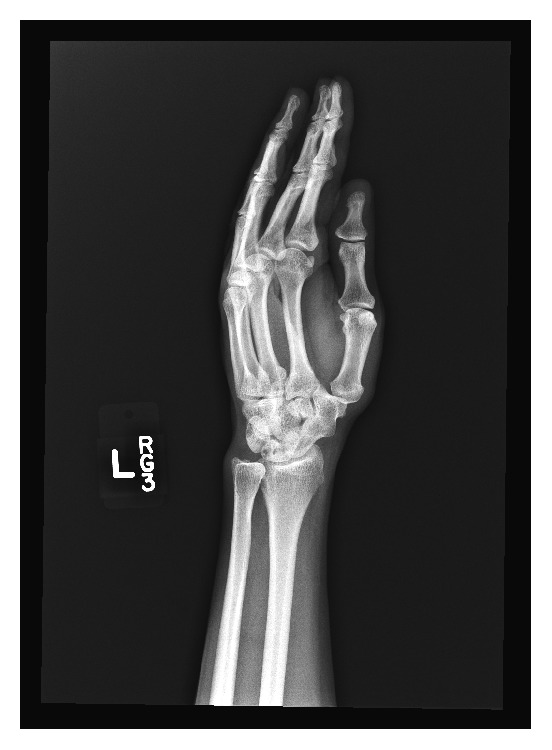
Oblique radiograph of the left wrist in patient 1 demonstrates osseous carpal coalition of the scaphoid and trapezium.
